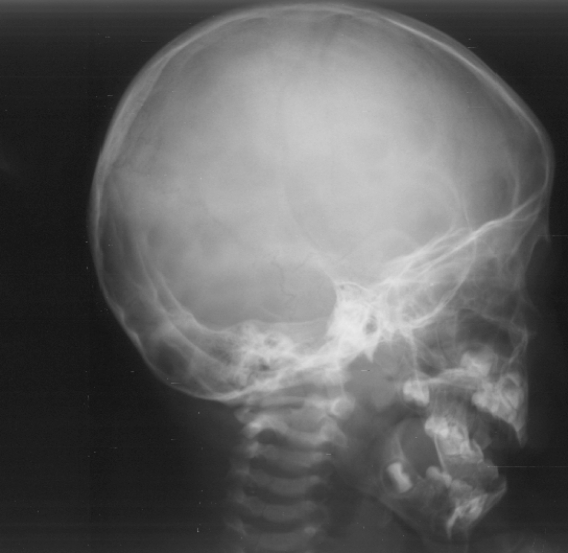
Lateral skull radiograph showed significant hyperostosis of the skull base associated with total sclerosis of the lambdoid sutures and partial sclerosis of the coronal sutures respectively. The anteroposterior length of both base and cranium are relatively shorter than that of the facial height.
